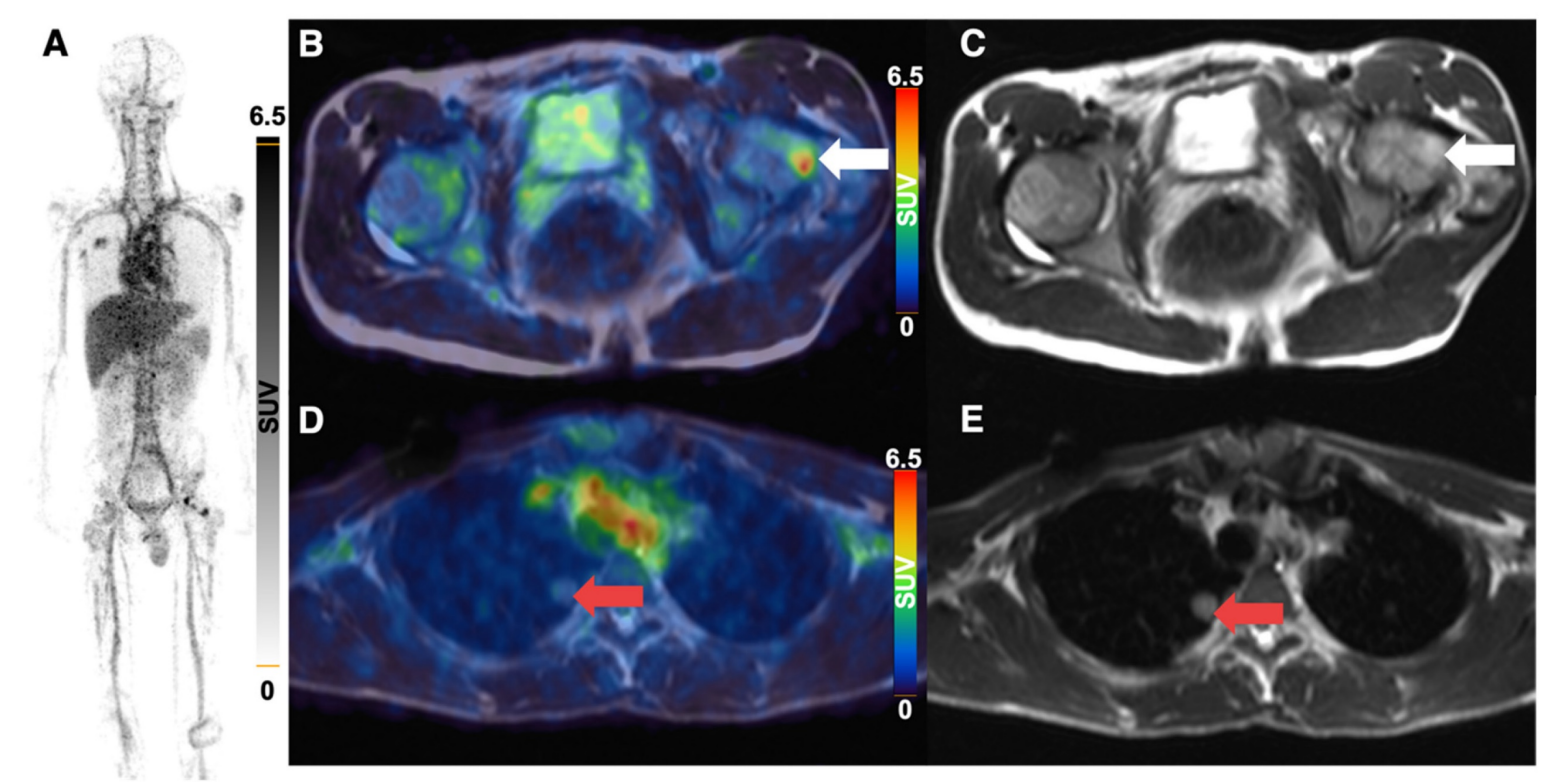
^64^Cu-GD2 PET/MRI of a 18 year old male patient with metastasized osteosarcoma (ID6). Maximum intensity projection of PET images on day 1 (A), transaxial representation of fused PET/MRI (B, D) as well as MRI (T2 HASTE sequence) (C, E) are shown. A ^64^Cu-GD2 positive bone metastasis (white arrow) was found in the left femur with a SUVmax of 8.5 (B, C). A lung metastasis (red arrow) demonstrates no significant uptake (D, E).
